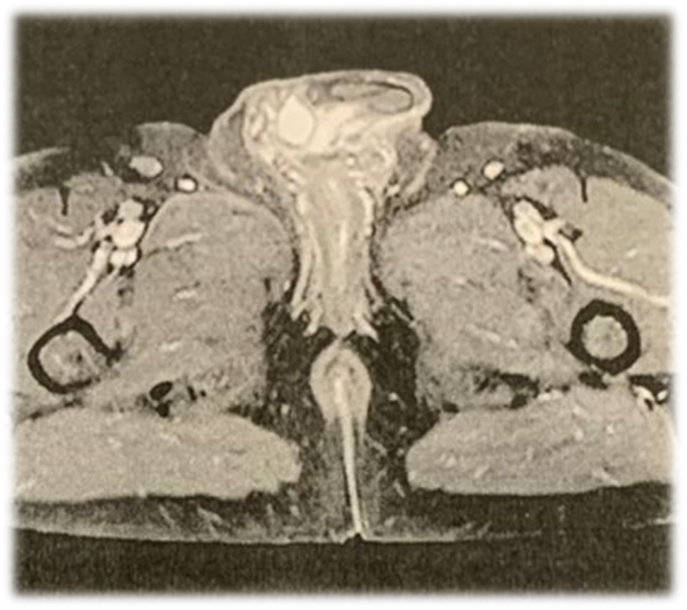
MRI taken 3 months after surgery that shows a low T1 high T2 sharp border enhancing 21*16 mm right penile root mass near the corpus spongiosum. Recurrence of known patient pathology (LGFMS) was suggested.
